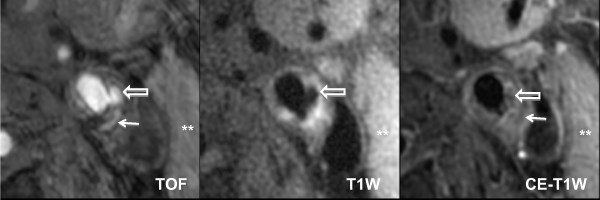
Magnetic Resonance Images of the Ruptured Carotid Plaque. The bright-blood 3D TOF image shows a distinct disruption of the fibrous cap (block arrow), corresponding to the hypointense juxtaluminal region on T1W and CE-T1W images. The hyperintense signal present in the necrotic core adjacent to the rupture (arrow) indicates presence of intraplaque hemorrhage inside of a lipid-rich necrotic core (thin arrow on CE-T1W image). Asterisks are placed on the sternocleidomastoid muscle, which is used to determine plaque signal intensities. The scan was acquired on a 3.0-T GE Signa Excite along with the use of a 4-element phased-array carotid coil.
